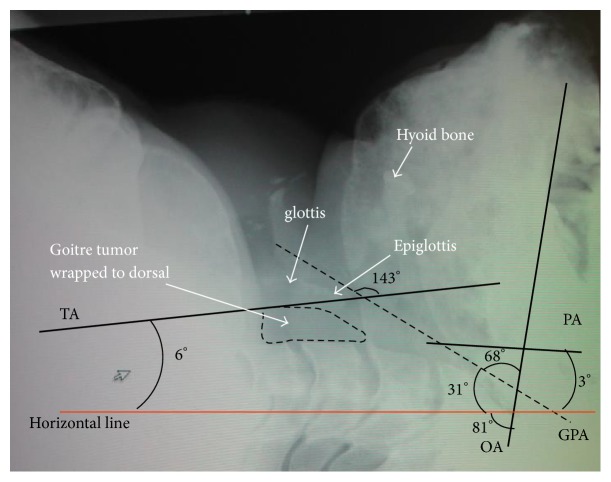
Preoperative lateral neck X-ray with the head resting on the bed in a neutral position. X-ray shows short neck with limited extension. The glottis is elevated by the dorsally located goitre. OA: oral axis, PA: pharyngeal axis, TA: tracheal axis, and GPA: glottis- pharyngeal axis.
